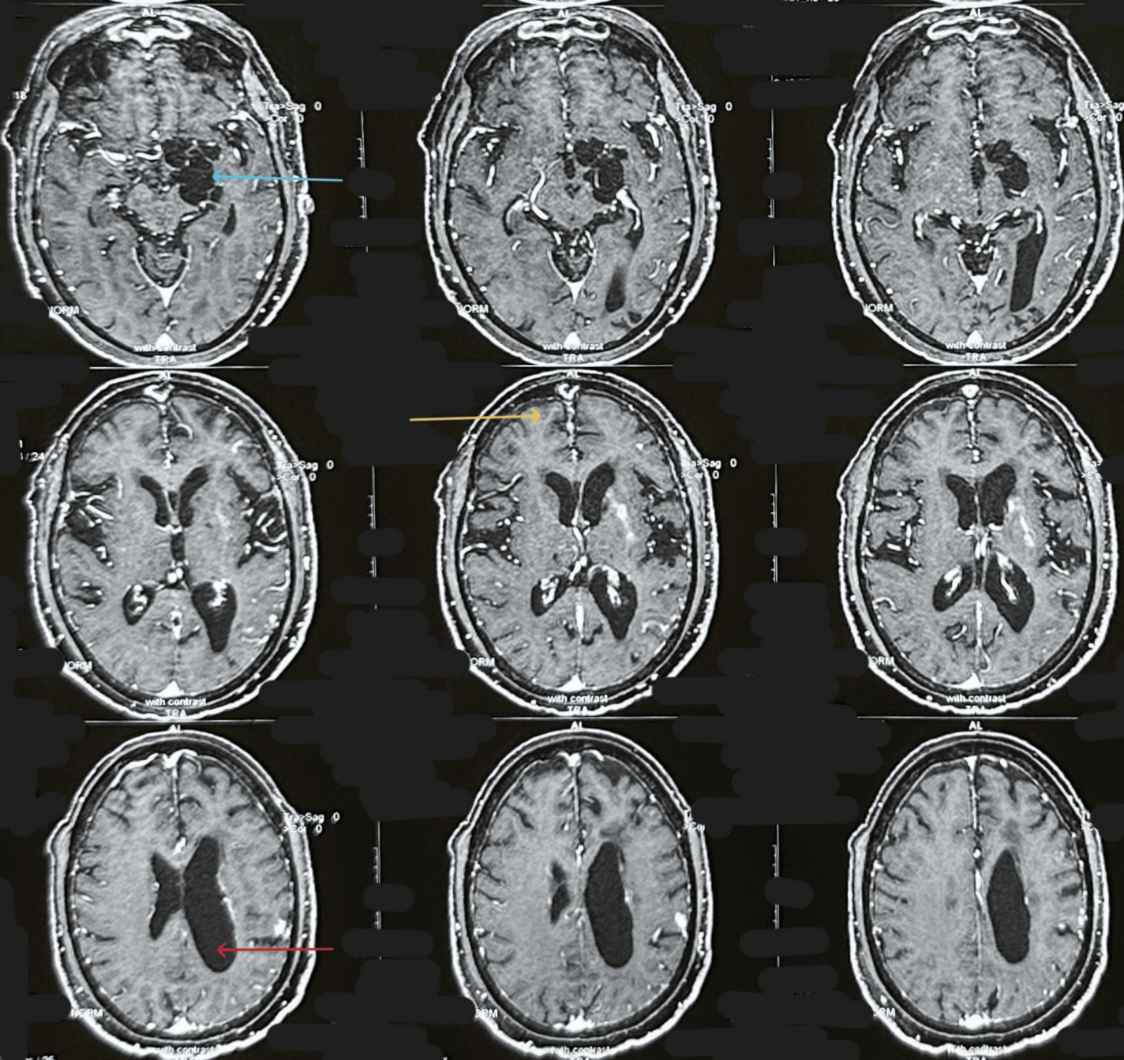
Contrast-enhanced MRI showing vesicular stage parenchymal neurocysticercosis in the right frontal lobe, racemose neurocysticercosis in the left crural cistern, and obstructive hydrocephalus of the left lateral ventricle The blue arrow indicates racemose neurocysticercosis; the yellow arrow highlights parenchymal neurocysticercosis in the vesicular stage with ring-enhancing lesions and eccentric scolex; the red arrow points to features of obstructive hydrocephalus caused by multiloculated cystic lesions.
